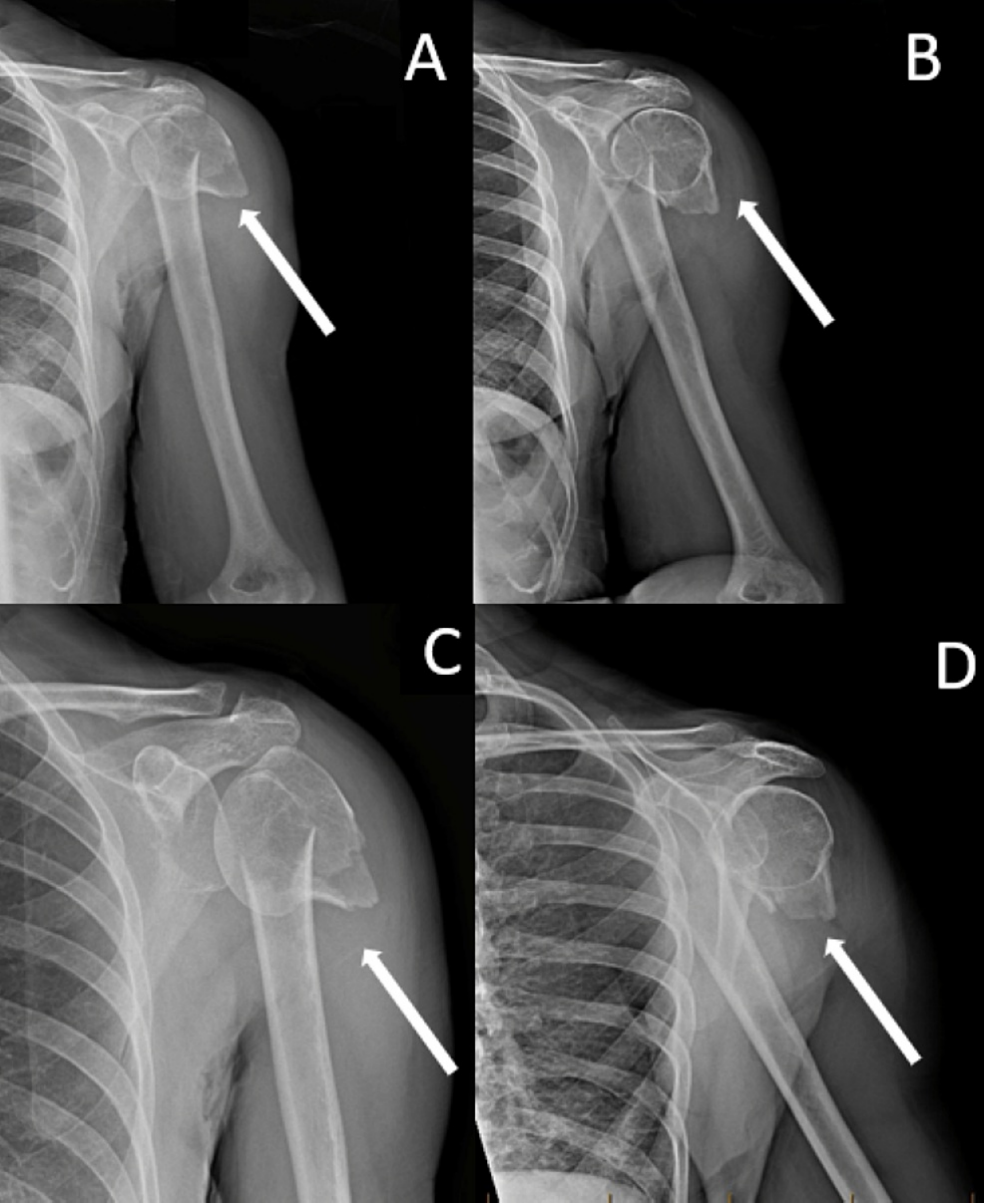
Anteroposterior and Lateral X-Ray Views of the Left Humerus and Shoulder Anteroposterior (AP) (A) and lateral (B) x-ray views of the left humerus and AP (C) and lateral (D) x-ray views of the left shoulder demonstrate a two-part left proximal humeral neck fracture with severe displacement and angulation between the proximal humeral head and distal humeral fragments.
